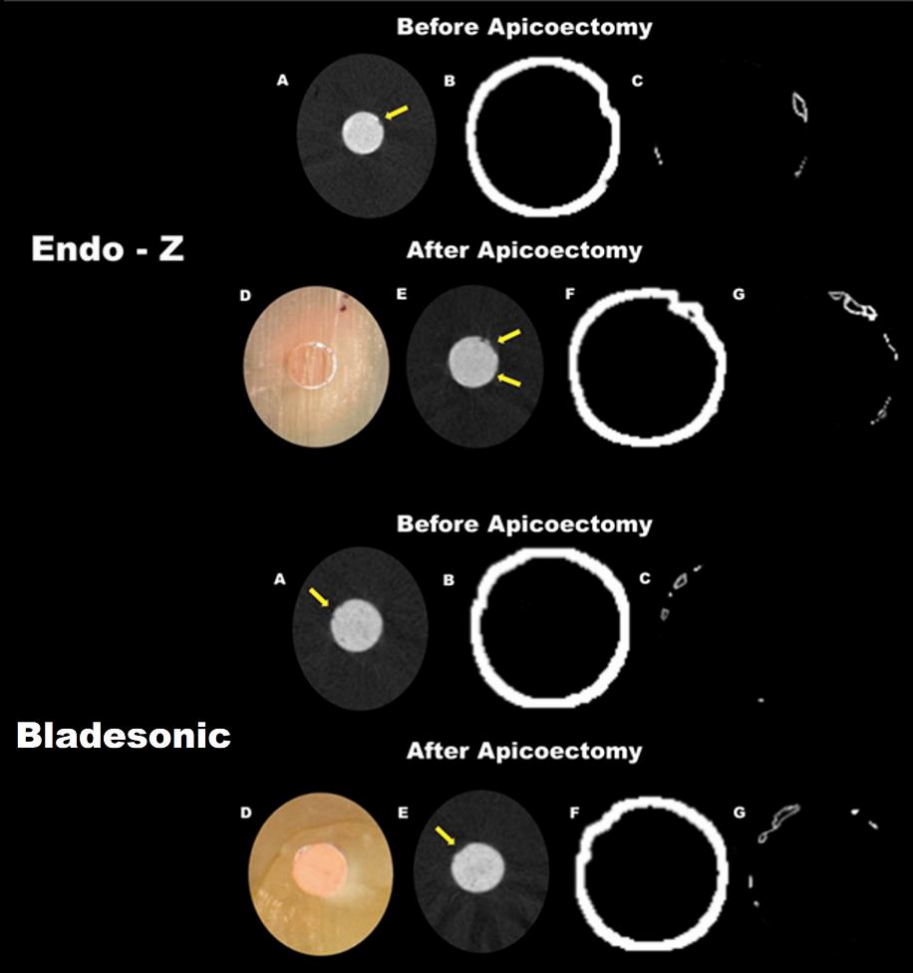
Representative images using CTAn software before and after apicoectomy using different devices. Root canals obturated with Bio-C Sealer (A and E), the yellow arrows point to the voids at the material/dentin interface; Images after apicoectomy using Endo-Z bur or Bladesonic ultrasonic tip, respectively (D); Volume interface: material/dentin (B and F); Presence of voids at the material/dentin interface (C and G).
